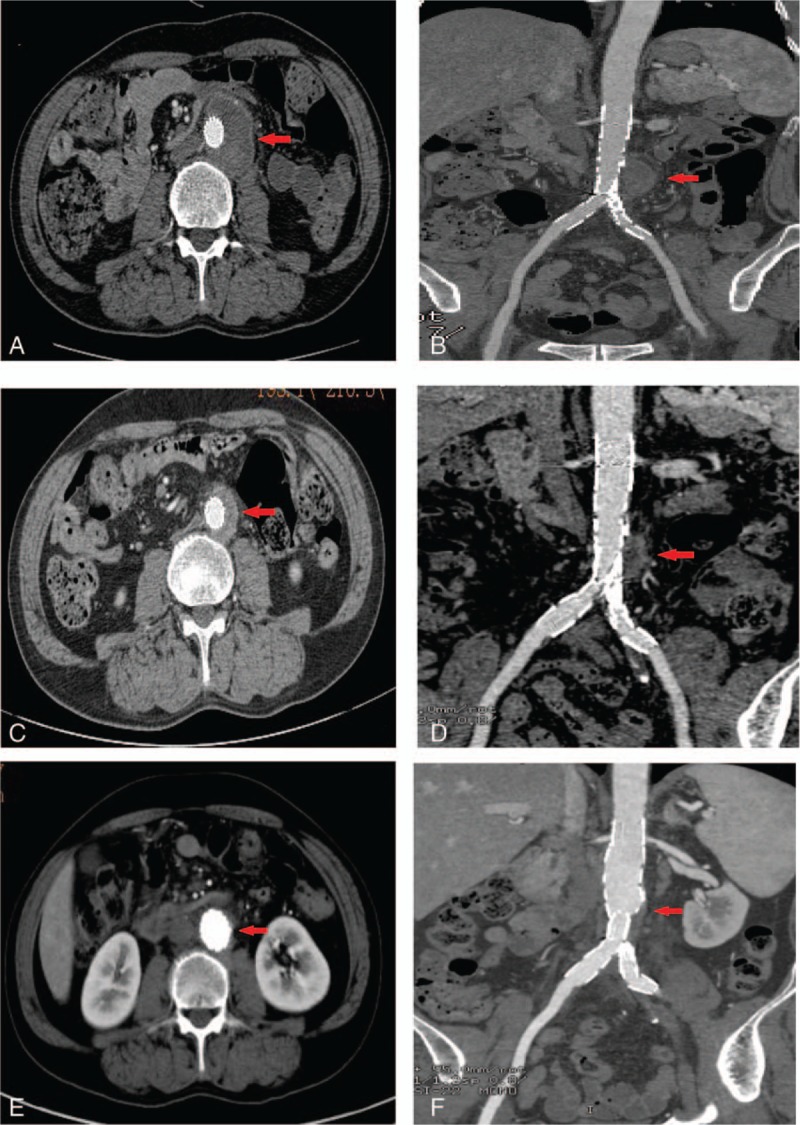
Three-dimensional CTA imaging shows complete isolation and shrinking of the abdominal aortic pseudoaneurysm. (A, B) One week after performing endovascular repair, there was a complete isolation of the abdominal aortic pseudoaneurysm with no signs of an endoleak. (C, D) Fifty days after the operation, the abdominal aortic pseudoaneurysm had shrunk significantly. There were no signs of infection in the surrounding tissues. (E, F) Ten months after the operation, the abdominal aortic pseudoaneurysm had disappeared almost completely. Arrows indicate the original position of the abdominal aortic aneurysm. 3D-CTA = three-dimensional computed tomography angiography.
